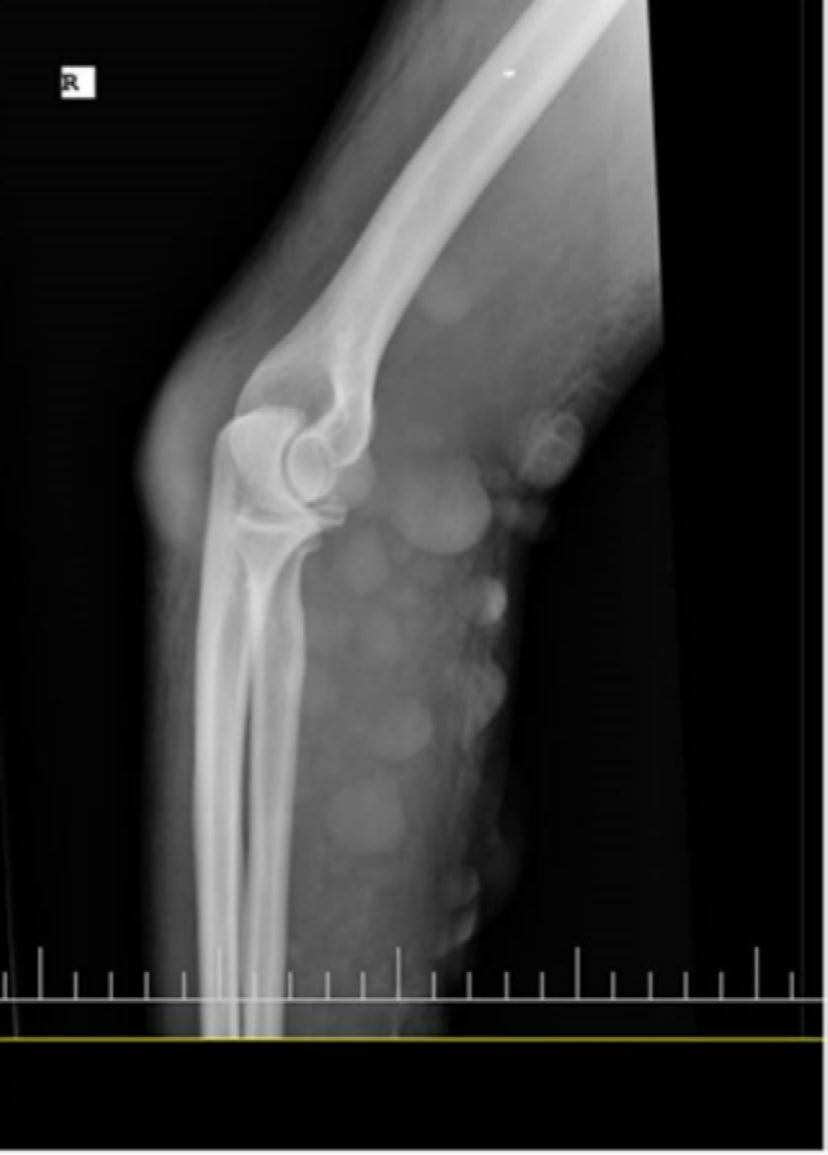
Radiography of the right upper limb demonstrated no evidence of fracture.
